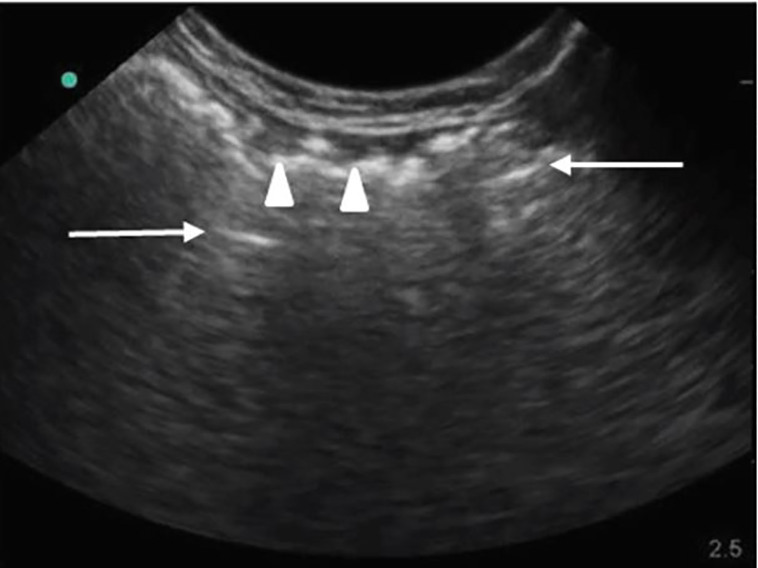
Point-of-care ultrasound of the neck done by the emergency physician, showing hyperechoic soft-tissue emphysema (arrowheads) with posterior acoustic shadowing and reverberation artifacts (arrows).
